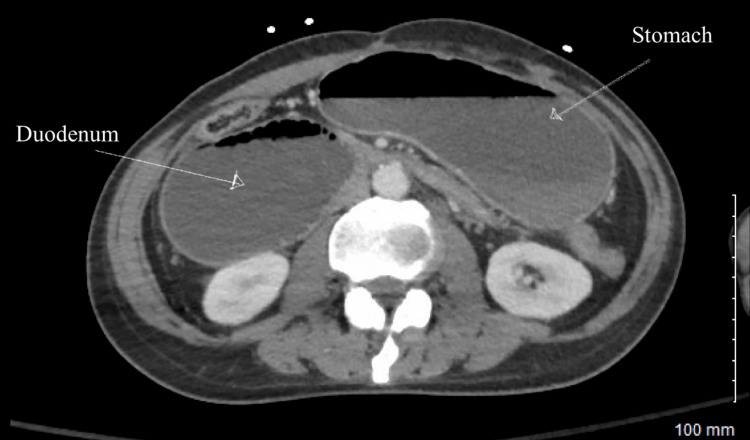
Axial computed tomography scan displaying the compressed duodenum and the dilated stomach In this image, the compressed duodenum and dilated stomach due to obstruction in gastric emptying were captured. This suggests that the patient exhibits characteristics associated with superior mesenteric artery (SMA) syndrome.
